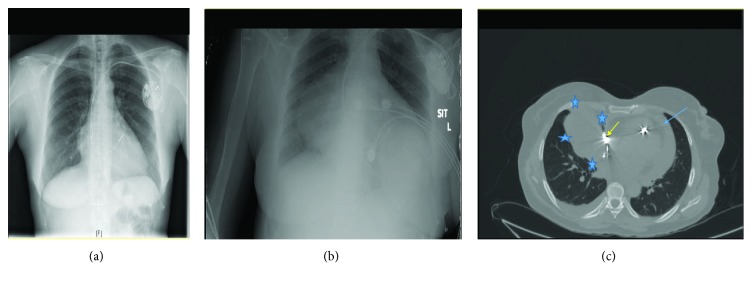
(a) Chest X-ray immediately following pacemaker insertion procedure showing normal right atrial contour with no opacification in the right lung field. (b) Chest X-ray showing opacity in the right middle lobe on admission. (c) Computed tomography showed a thickened pericardium and pericardial effusion (blue arrow), pericardial cyst (blue stars), and the pacemaker electrode lining the epicardial surface of the right ventricle (yellow arrow).
